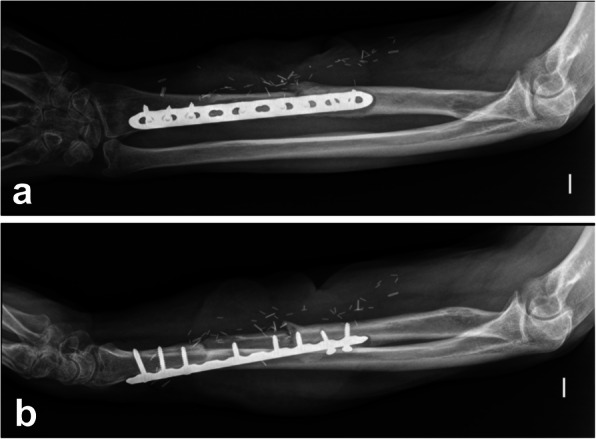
Postero-anterior (a) and lateral (b) radiographs of a chronic internal fixation related infection due to *Pseudomonas aeruginosa* following a Gustilo III-C open fracture of the radial diaphysis in a 62-year-old male. Note the radiolucent line surrounding the plate and the screw backout
